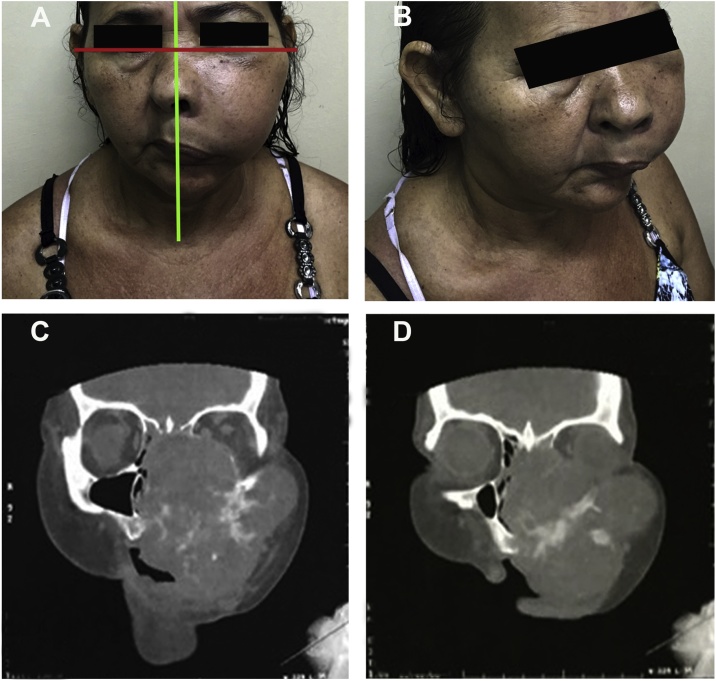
Clinical extraoral photograph at the beginning of treatment and Pre-treatment multislice computed tomography. In 1A is observed facial asymmetry. In 1B is observed in the maxilla region between the zygomatic arch and the left labial commissure the extent of the tumor that promotes dystopia and nasolabial fold deletion with right nose displacement. In 1C and 1D is observed a large expansive hypodense image involving the middle third of the face.
